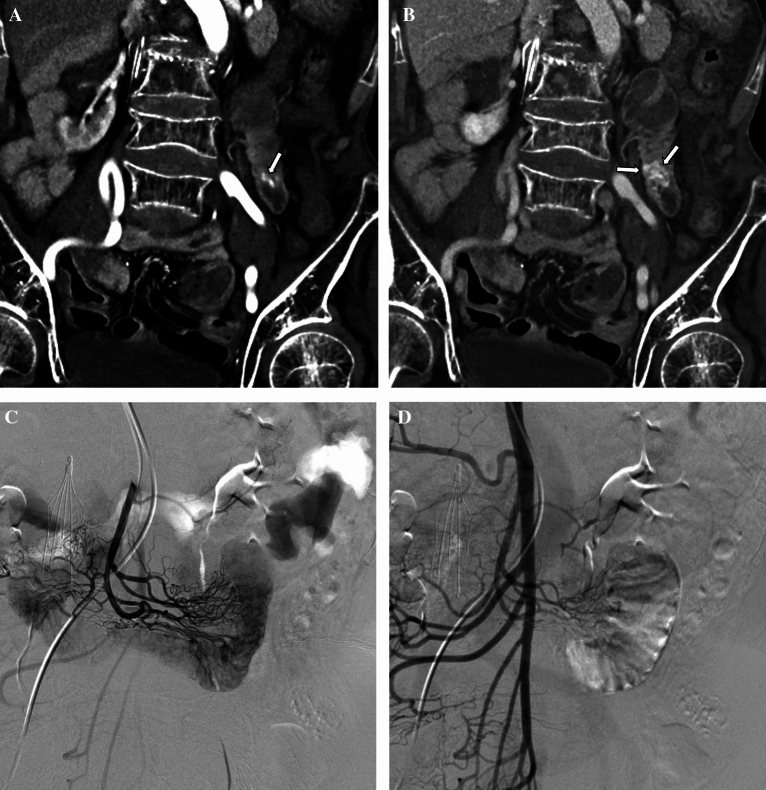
A 63-year-old woman with underlying Behçet’s disease presented with hematochezia 1 day prior (Patient 3). (a,b) Arterial (a) and venous (b) phases of computed tomography show active bleeding (arrows) in the jejunum. (c) Selective angiography through the superior mesenteric artery shows no definite bleeding focus at the jejunum matching the bleeding site indicated by computed tomography. These jejunal branches were embolized with quick-soluble gelatin sponge particles (350–560 µm) (not shown). (d) The embolized jejunal branches were not visible via completion superior mesenteric arteriography. She had no rebleeding during 22 months of follow-up.
